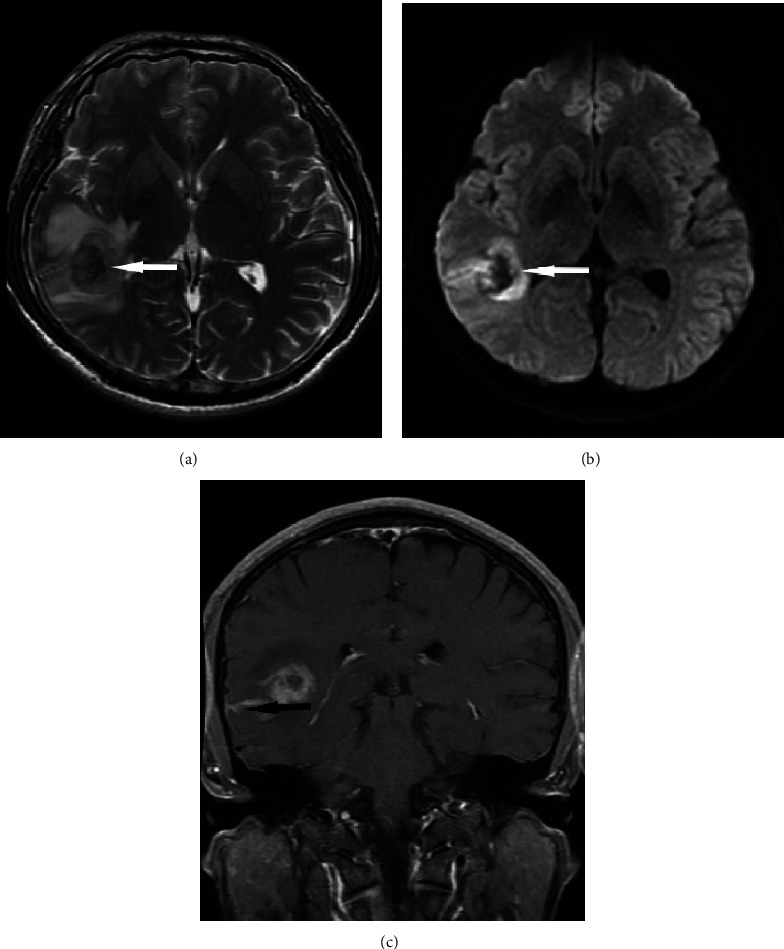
The patient shown was a 41-year-old male patient with white matter eGBM in the right temporal lobe. The boundary of the lesion was unclear, and there was a large area of edema around it. The lesion was accompanied by hemorrhage. The lesion showed a low signal on (a, white arrow) T2WI and (b, white arrow) DWI. On a contrast-enhanced scan, the lesion showed inhomogeneous enhancement and invaded the adjacent meninges (c, black arrow).
